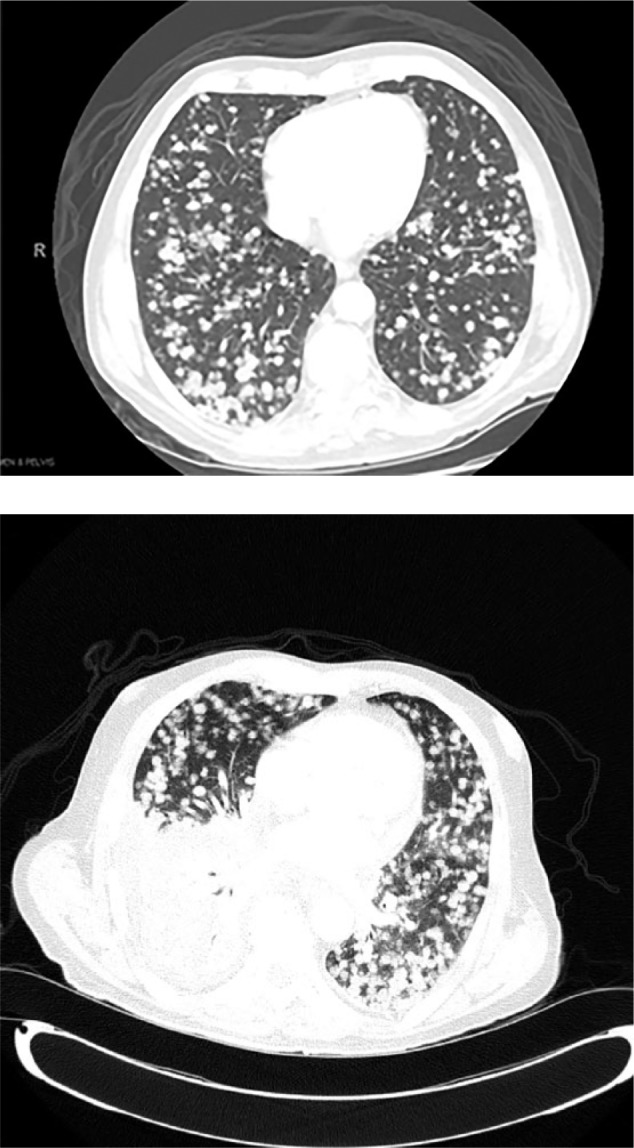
Before and after CT scans of the chest with contrast showing pulmonary metastasis and newly.
